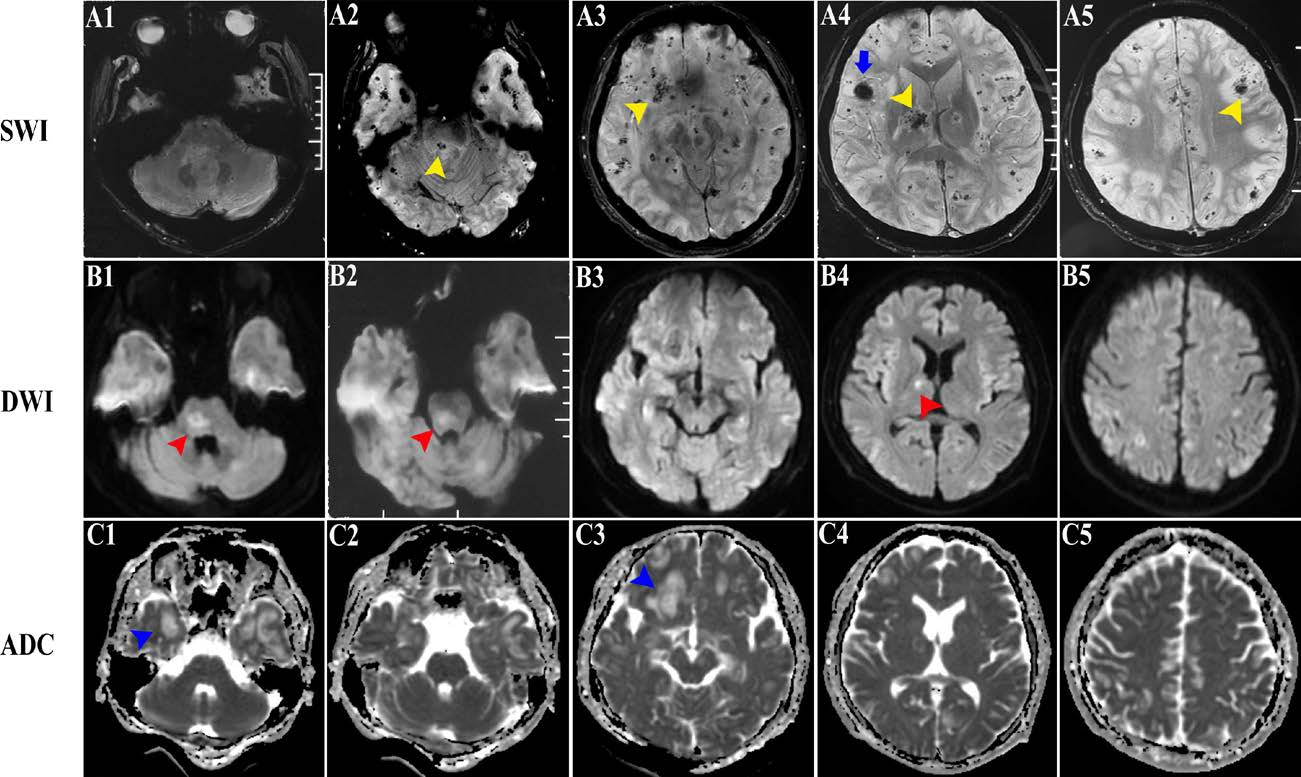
MR images of susceptibility-weighted image (SWI), diffusion-weighted image (DWI) and apparent diffusion coefficient (ADC) map on day 6. SWI present multiple extensive petechial hemorrhage in cortex, white matter, gray-white junction (yellow arrow) and a large intracerebral bleed in right frontal lobe (blue arrowhead). DWI and ADC axial sections reveal diffusion restriction in some lesions (red arrow), but in other lesions apparent diffusion coefficient increases (blue arrow).
